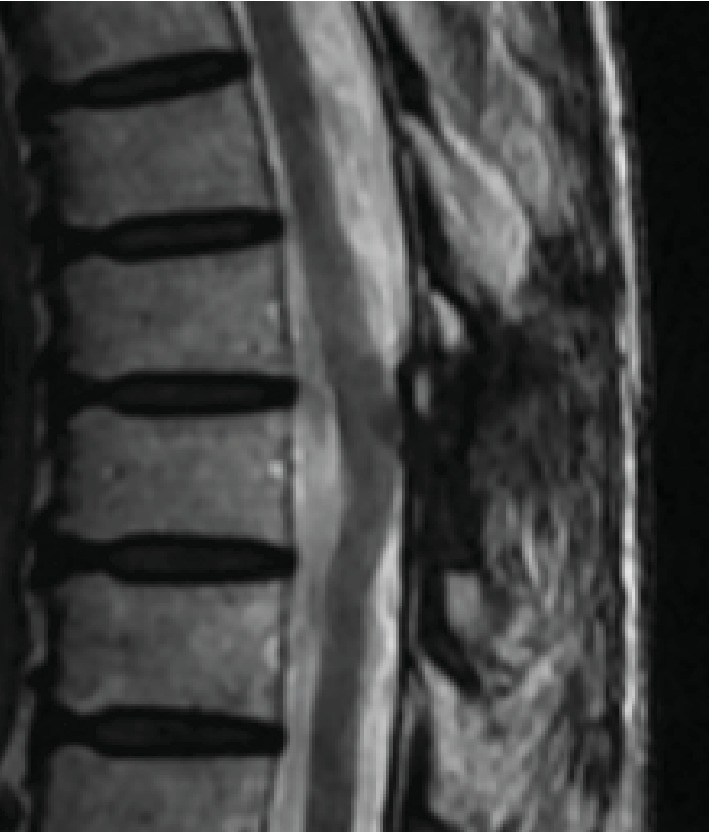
MRI of the thoracic spine showed no evidence of tumor recurrence three years after the surgery for intradural-extramedullary SFT/HPC.
